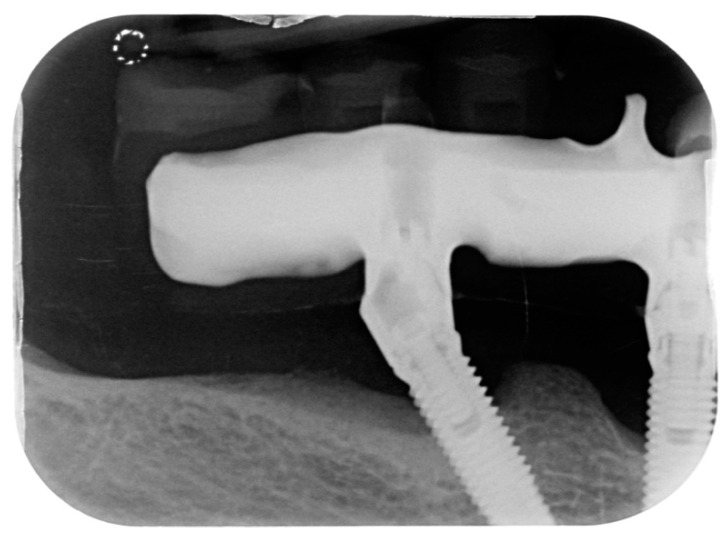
Periapical radiograph belonging to the only diabetic patient who developed peri-implantitis.
